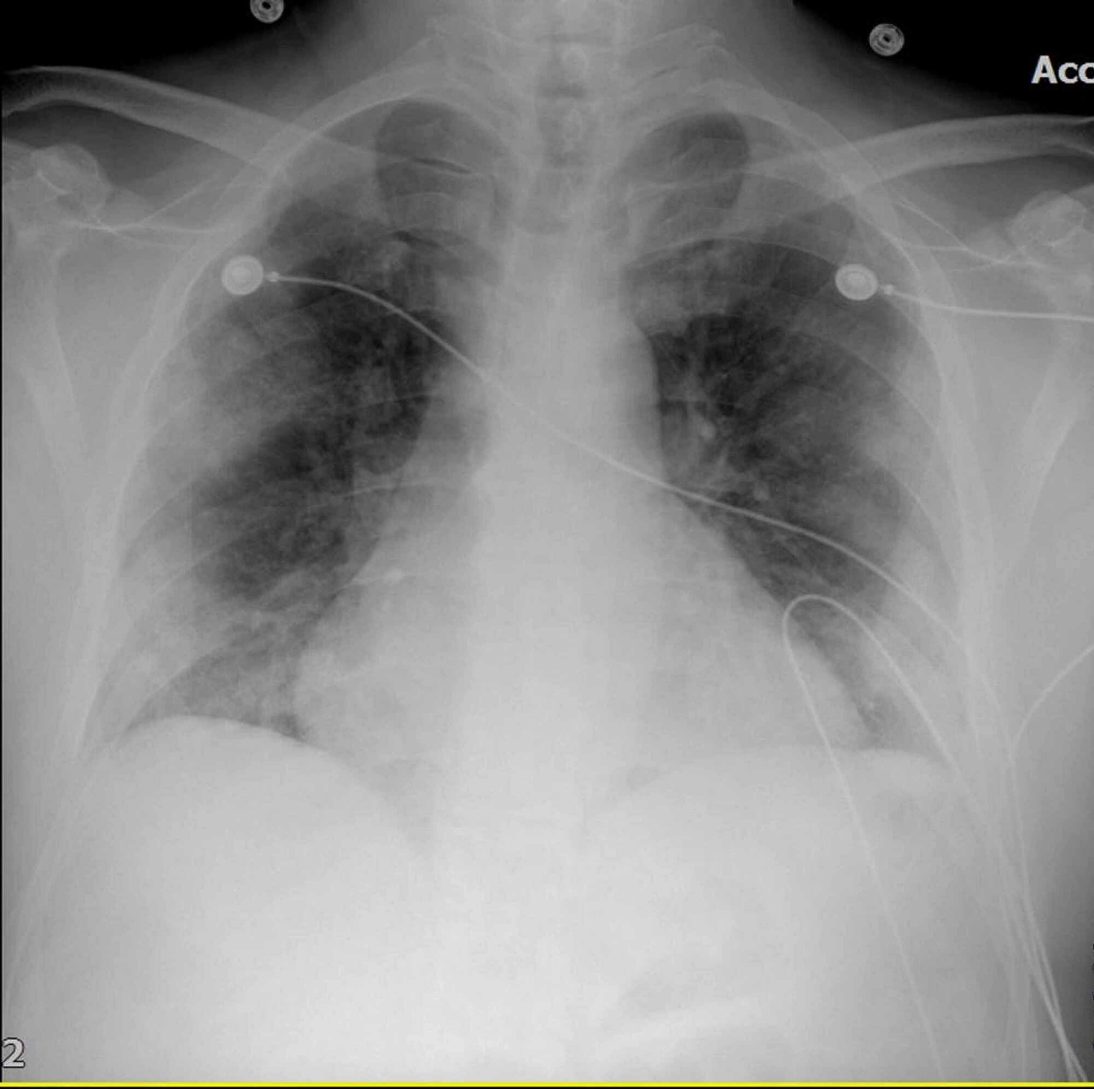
Chest X-ray showing bilateral ground glass infiltrate and COVID pneumonitis.
